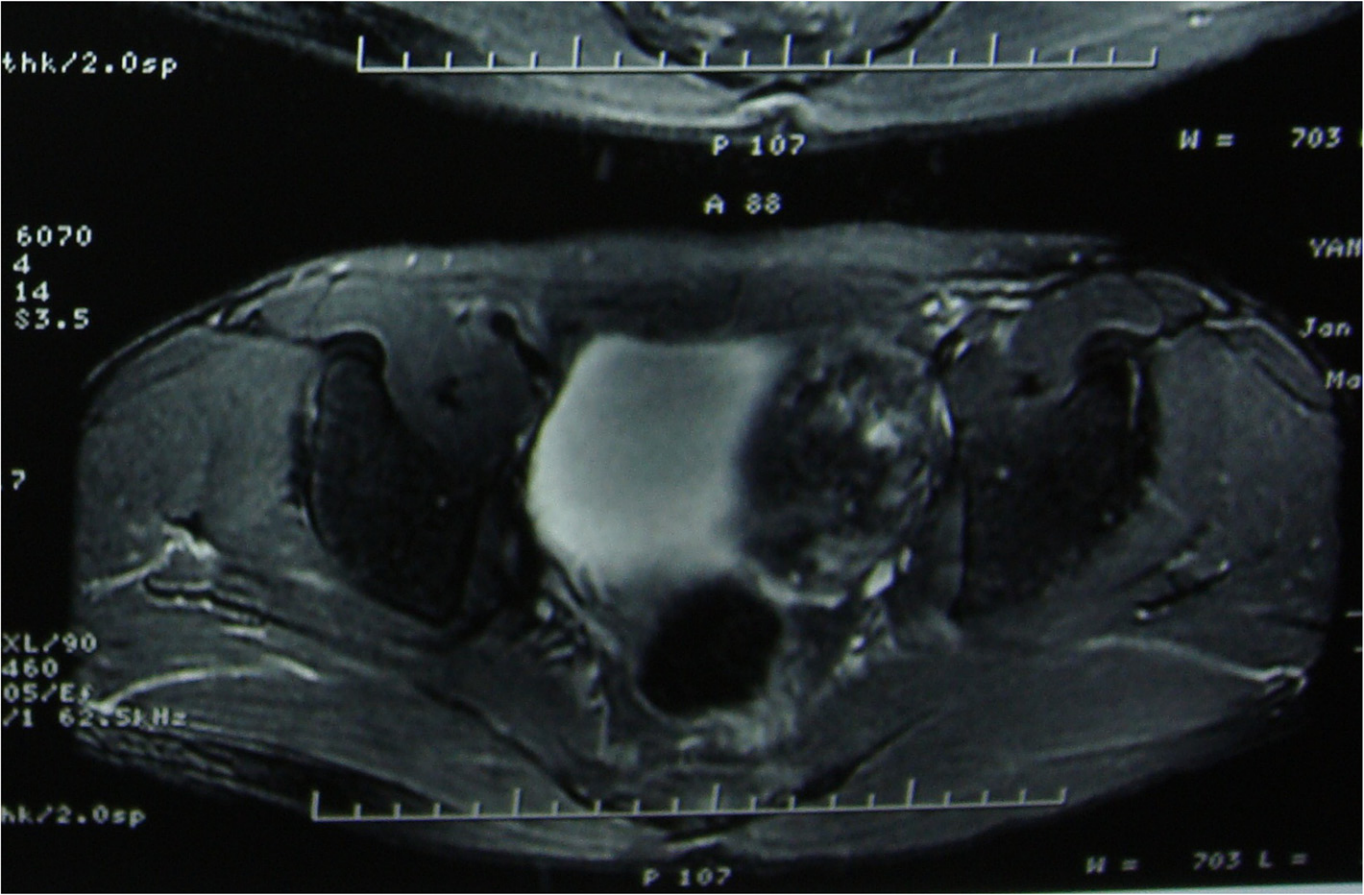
Magnetic resonance imaging (MRI): an abnormal signal mass in the left seminal vesicle.
